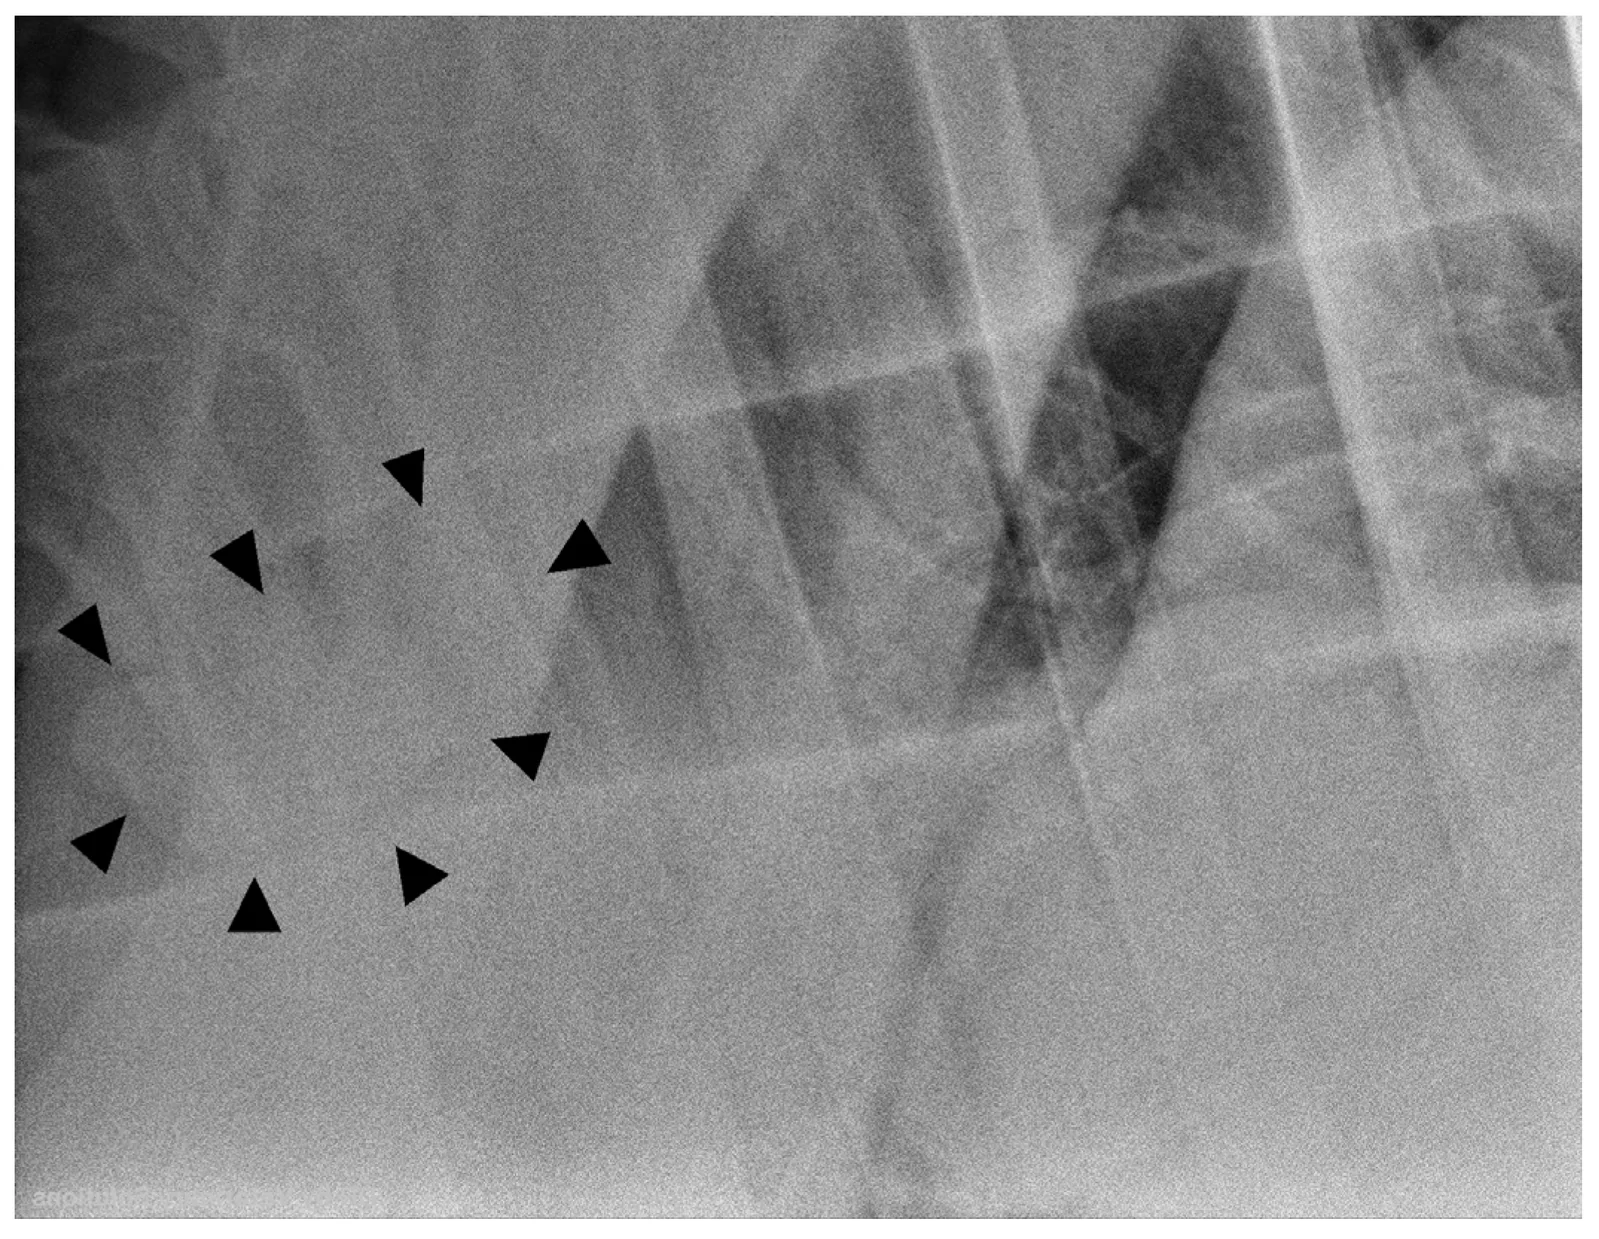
A pre-surgical radiograph of the trachea (with the caudal end to the right and the proximal end at the top) demonstrates a well-defined, round soft-tissue mass within the tracheal lumen at the level of the thoracic inlet, indicated by the black arrowheads.
